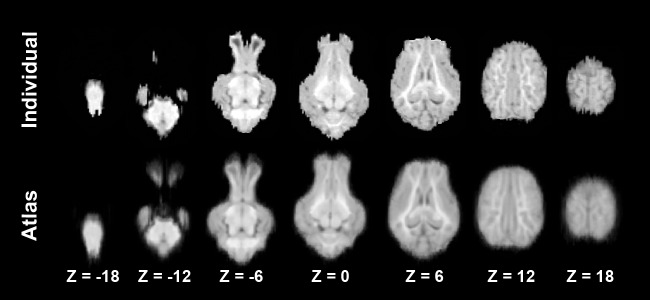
Comparison of an individual MRI data set and the atlas. The axial slices are from −18 mm to 18 mm in 6 mm increments. The top row is a representative individual case and the bottom row is the atlas.
